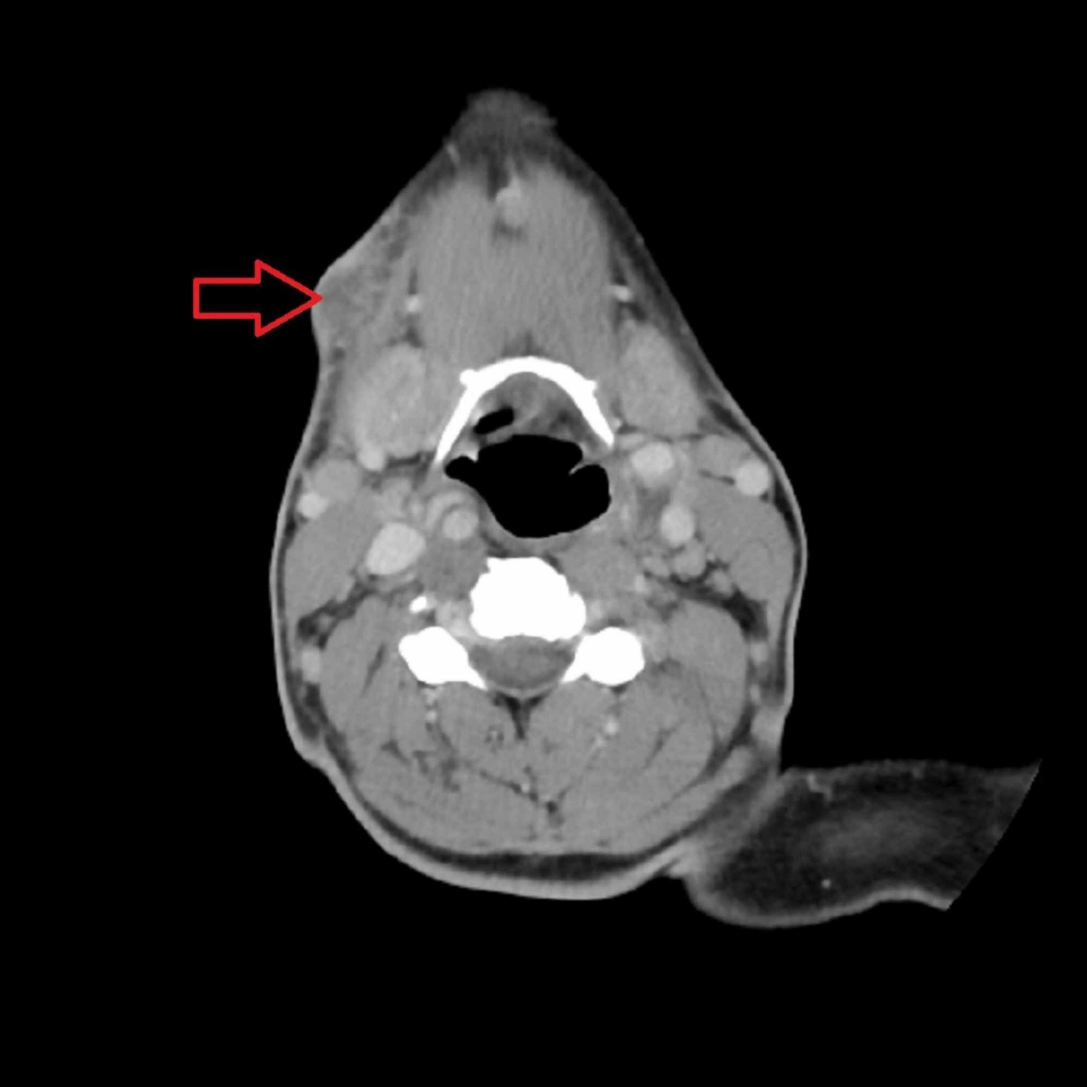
CT scan, showing the neck abscess in the anterior part on the right side.
